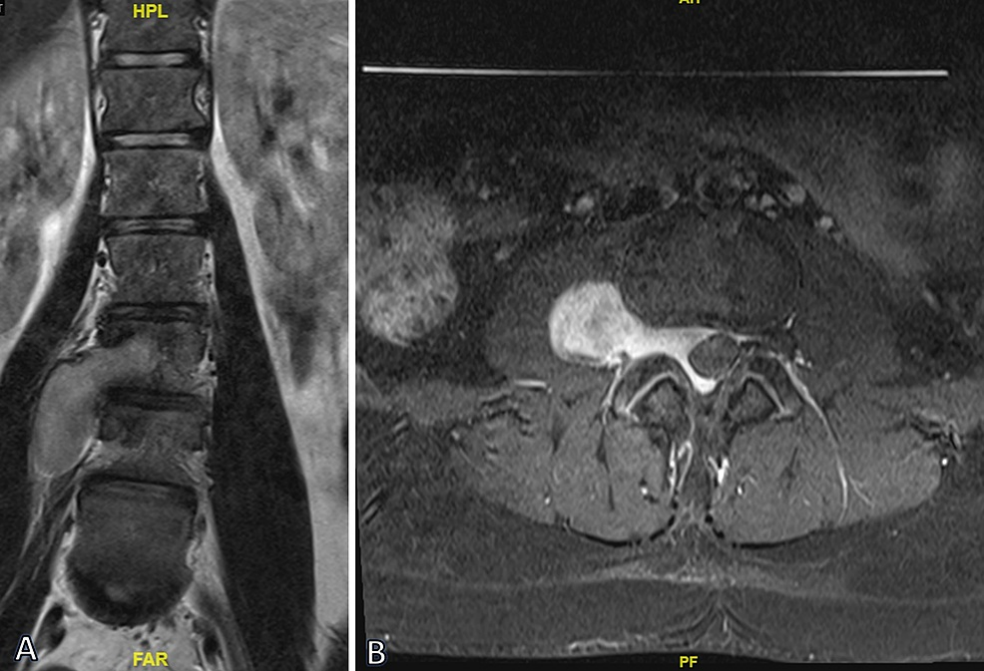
(A) Preoperative coronal T2-weighted MRI demonstrating the mass at the right L3-L4 level with extension into the right psoas muscle and (B) on postcontrast axial MRI with the same mass causing mild effacement of the thecal sac.
